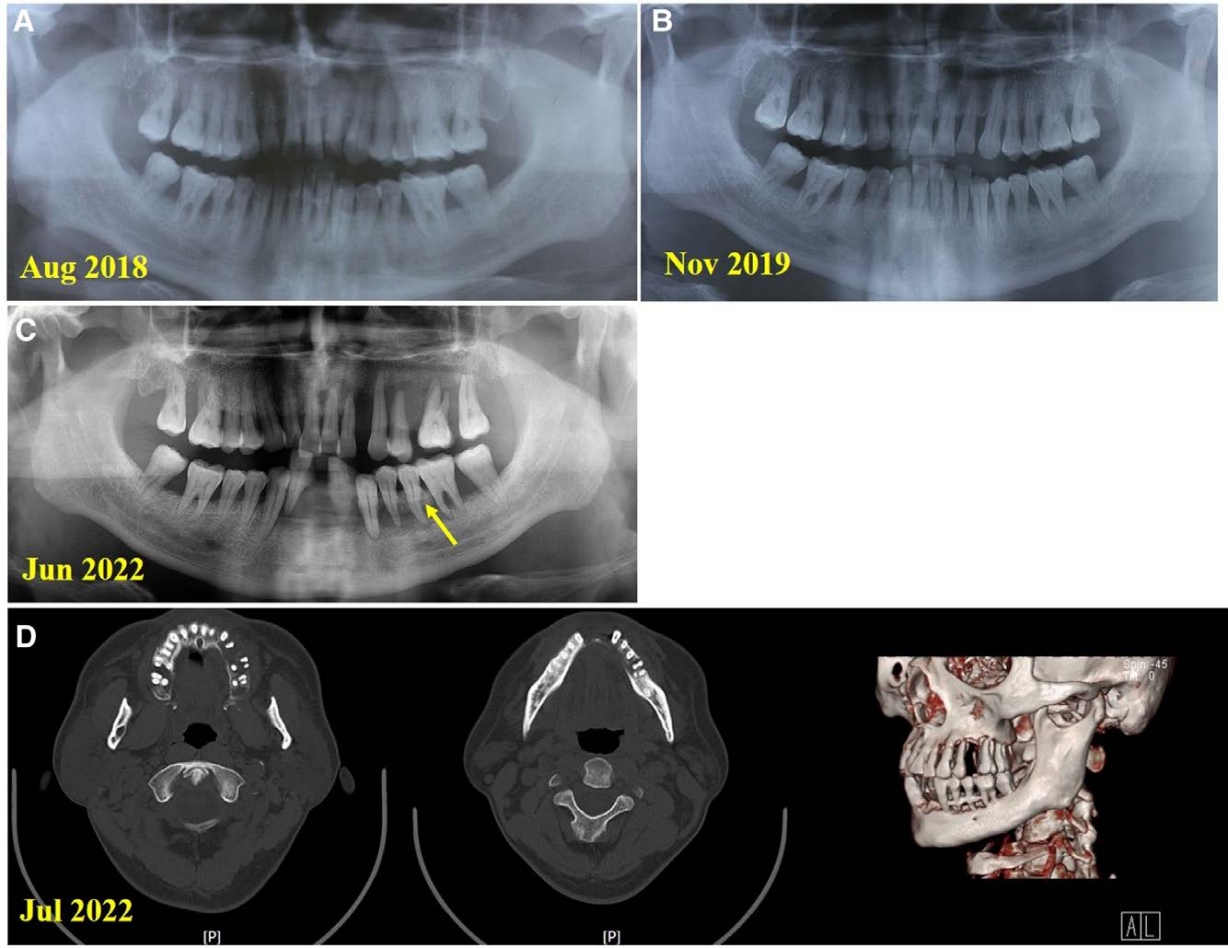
Orthopantomogram and spiral computed tomography images of the patient. (A, B) Images obtained at different times demonstrate rapidly progressing alveolar bone resorption and missing teeth. (C, D) Alveolar bone necrosis on teeth 33 to 35. Yellow arrow: Alveolar bone necrosis.
